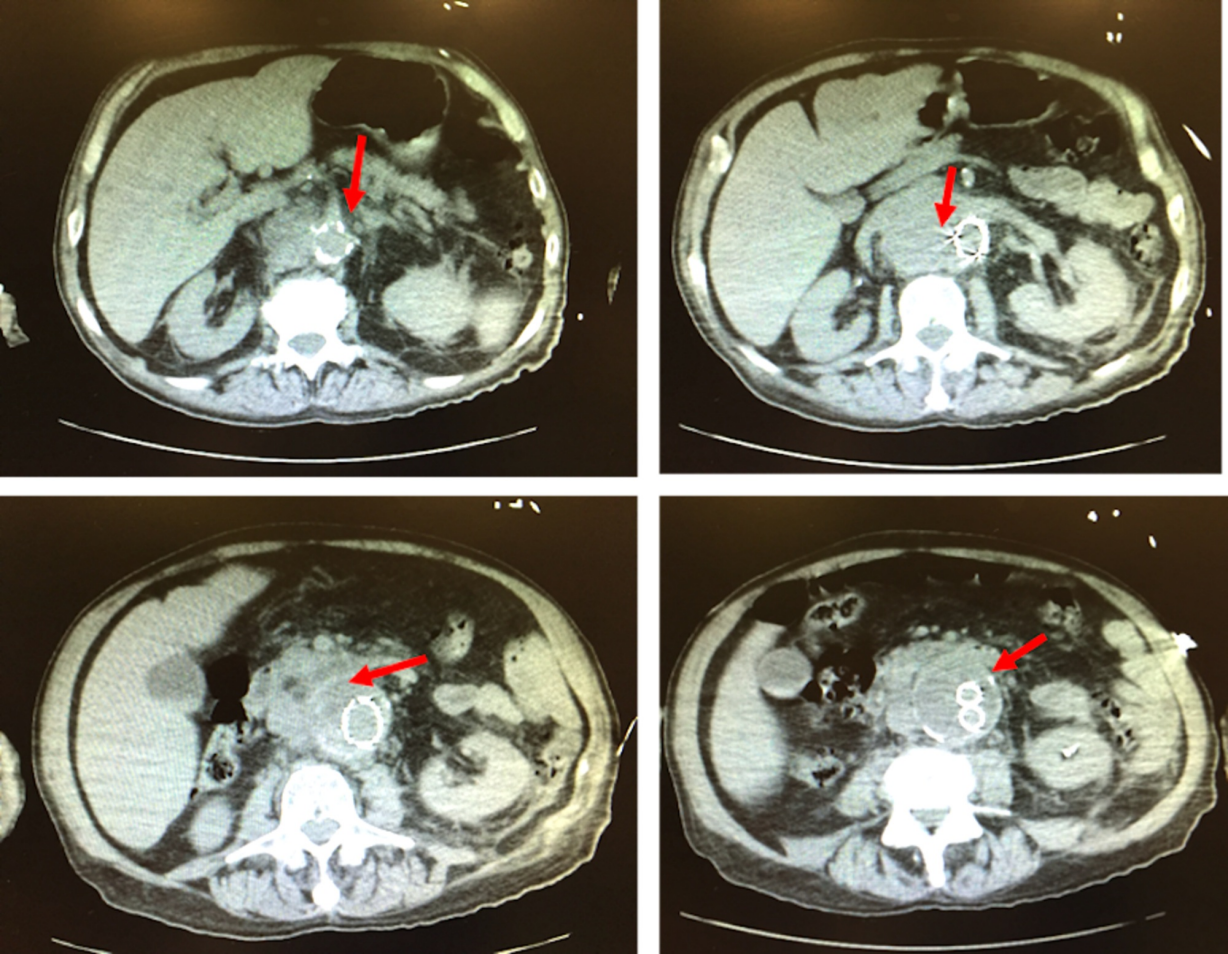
Noncontrast CT scan demonstrating false lumen of abdominal aortic aneurysm (red arrows).
